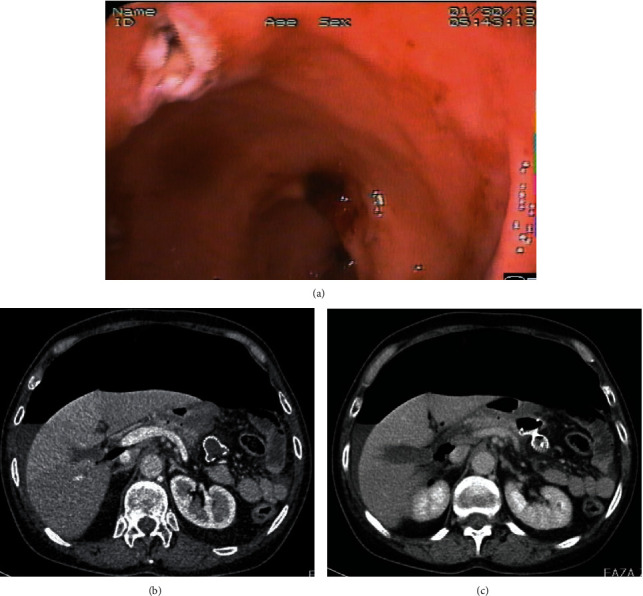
(a–c) Early proximal transmural stent migration. (a) The endoscopic image shows the transmural fistula without the stent. (b, c) Contrast-enhanced multiphase computed tomography image of the abdominal wall in a patient suffering from a perforation of the gastrointestinal tract due to early proximal dislocation of the transmural stent. (b, c) A large amount of air can be seen in the peritoneal cavity, as well as the dislodged transmural stent outside of the gastrointestinal lumen.
